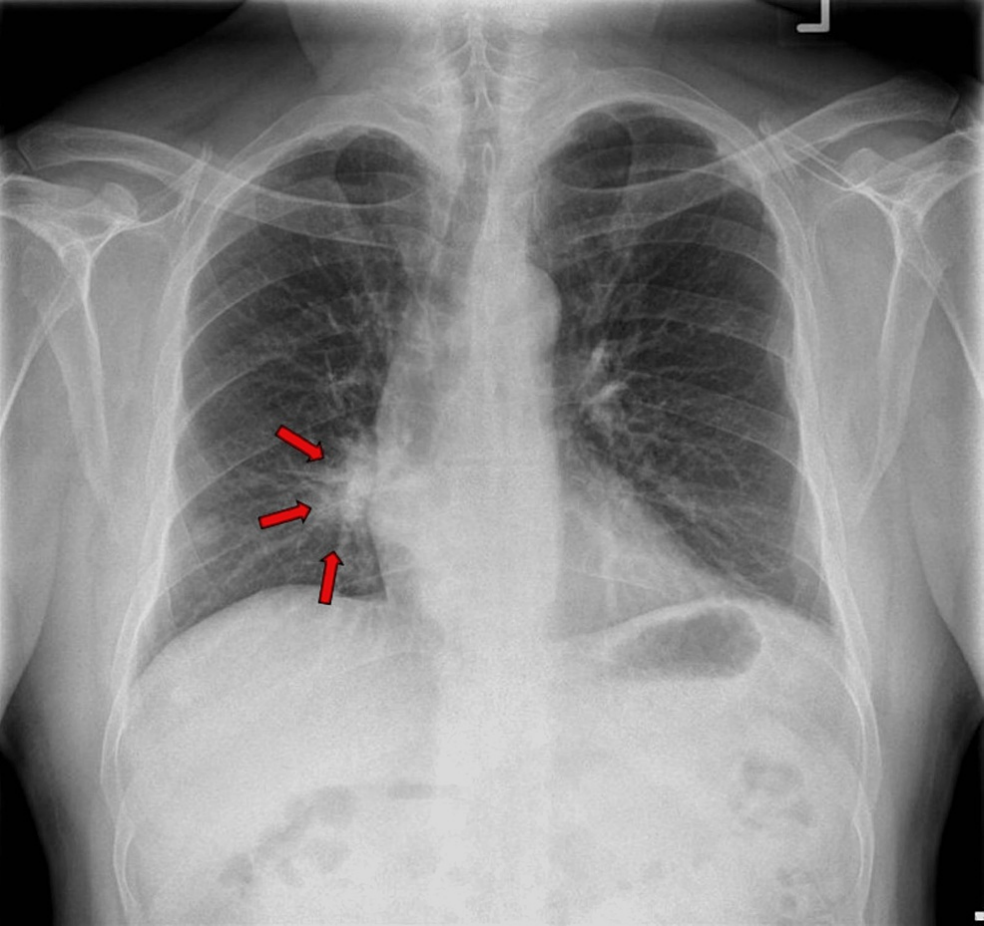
Chest radiograph after the patient complained of a cough and hemoptysis showing new right hilar mass-like consolidation.
